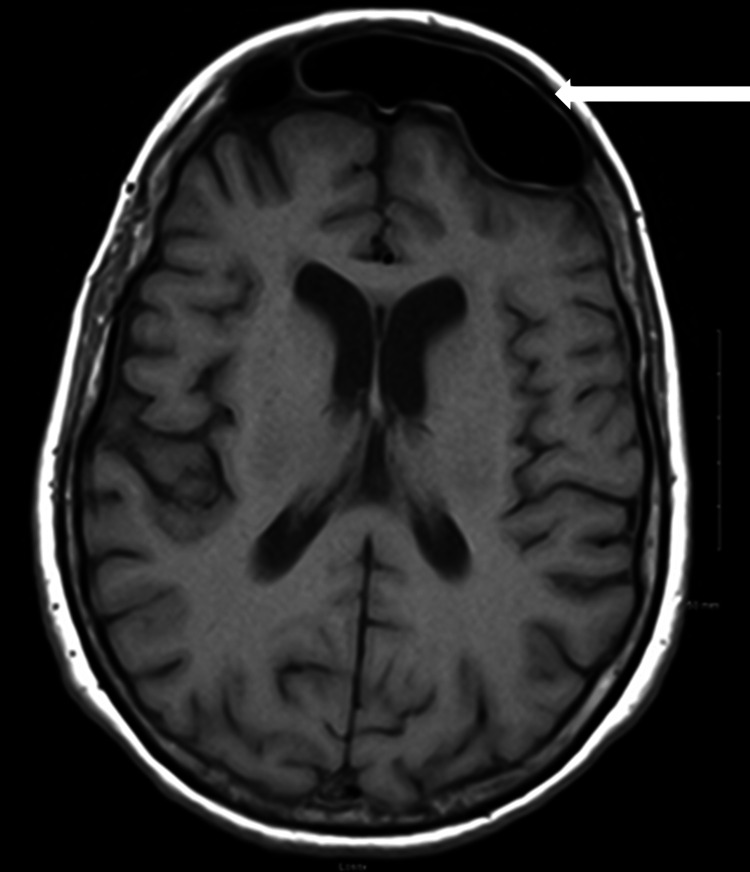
Postoperative axial T1 brain magnetic resonance imaging showing no residual extra-axial collection and post surgical changes of the sinuses with mild mucosal thickening (white arrow).
